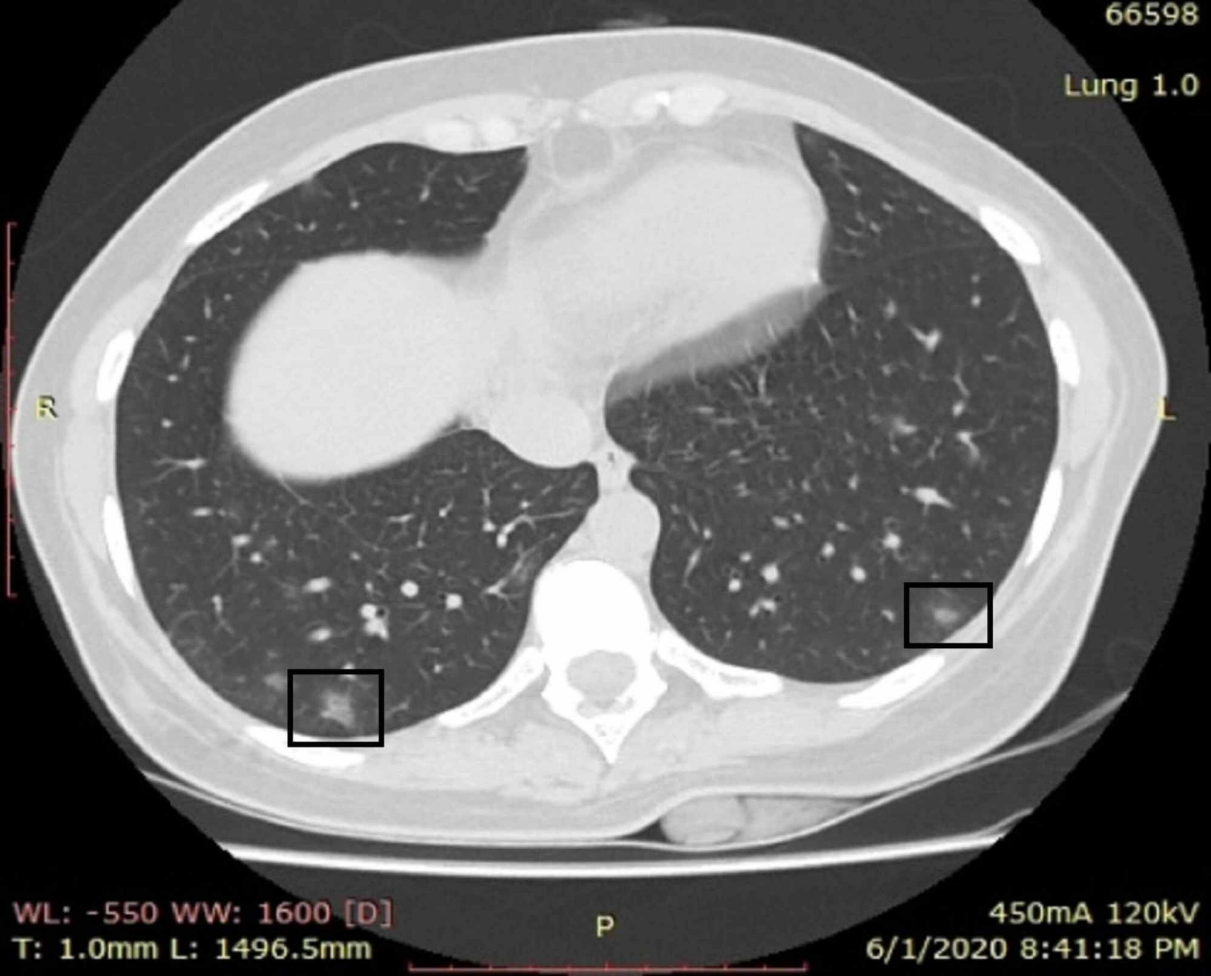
A young female patient with cough and fever who presented on the fifth day of symptoms CT shows small peripheral bilateral areas of ground-glass opacities in lower lobes (boxes). No area of consolidation was identified CT: computed tomography
